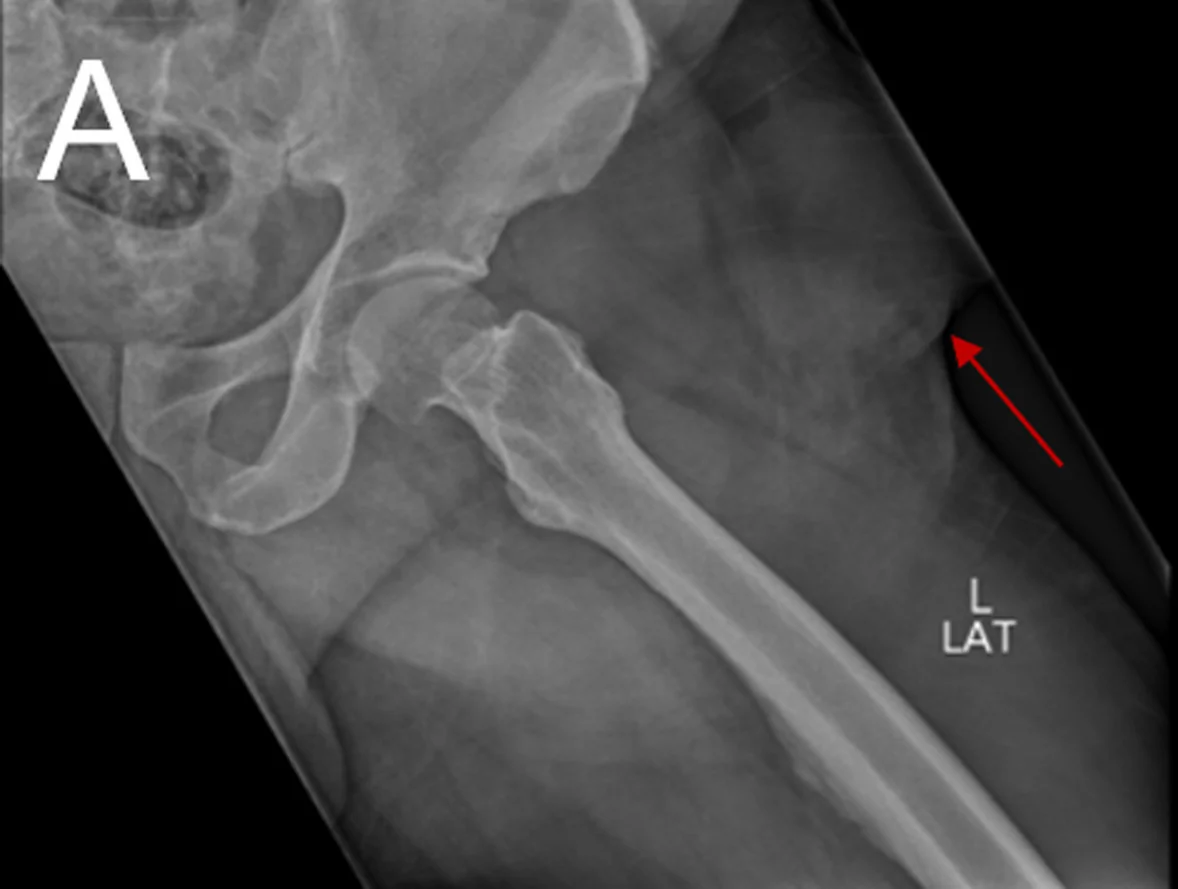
Pelvic radiograph demonstrating a soft tissue mass in the left gluteal region without calcification or underlying osseous involvement
